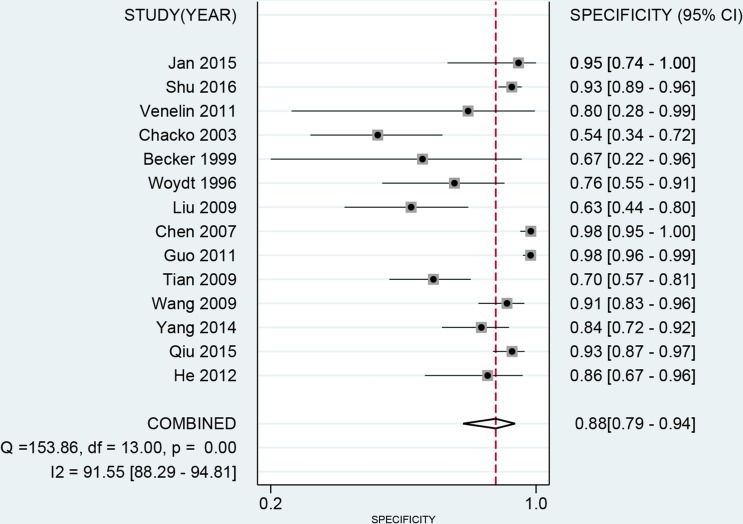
Forest plot of pooled specificity of intraoperative ultrasound for glioma residual
